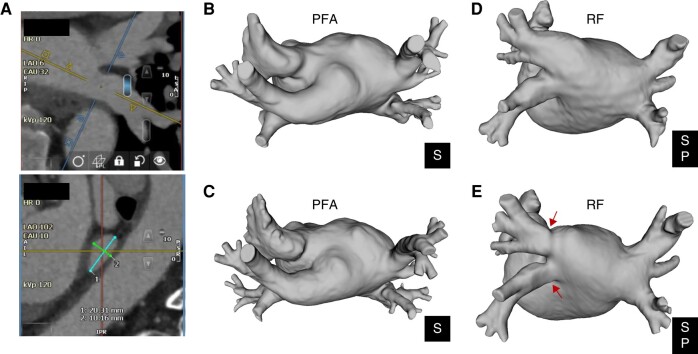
Example cardiac CT measurements showing the short and long diameter measurements (*A*) and images reconstructed in 3D renderings to show pre-procedural and 90-day pulmonary vein dimensions for a patient treated with PFA (*B*, *C*) and thermal (RF) ablation (*D*, *E*). P, posterior; PFA, pulsed field ablation; RF, radiofrequency; S, superior.
